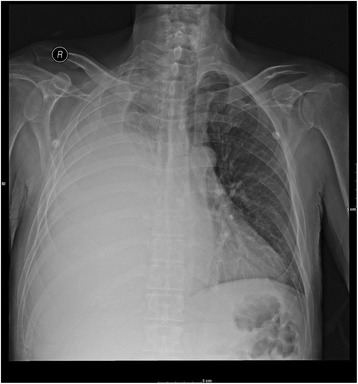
Chest X-ray shows almost complete opacification with a large right pleural effusion and consolidated right lower lobe
